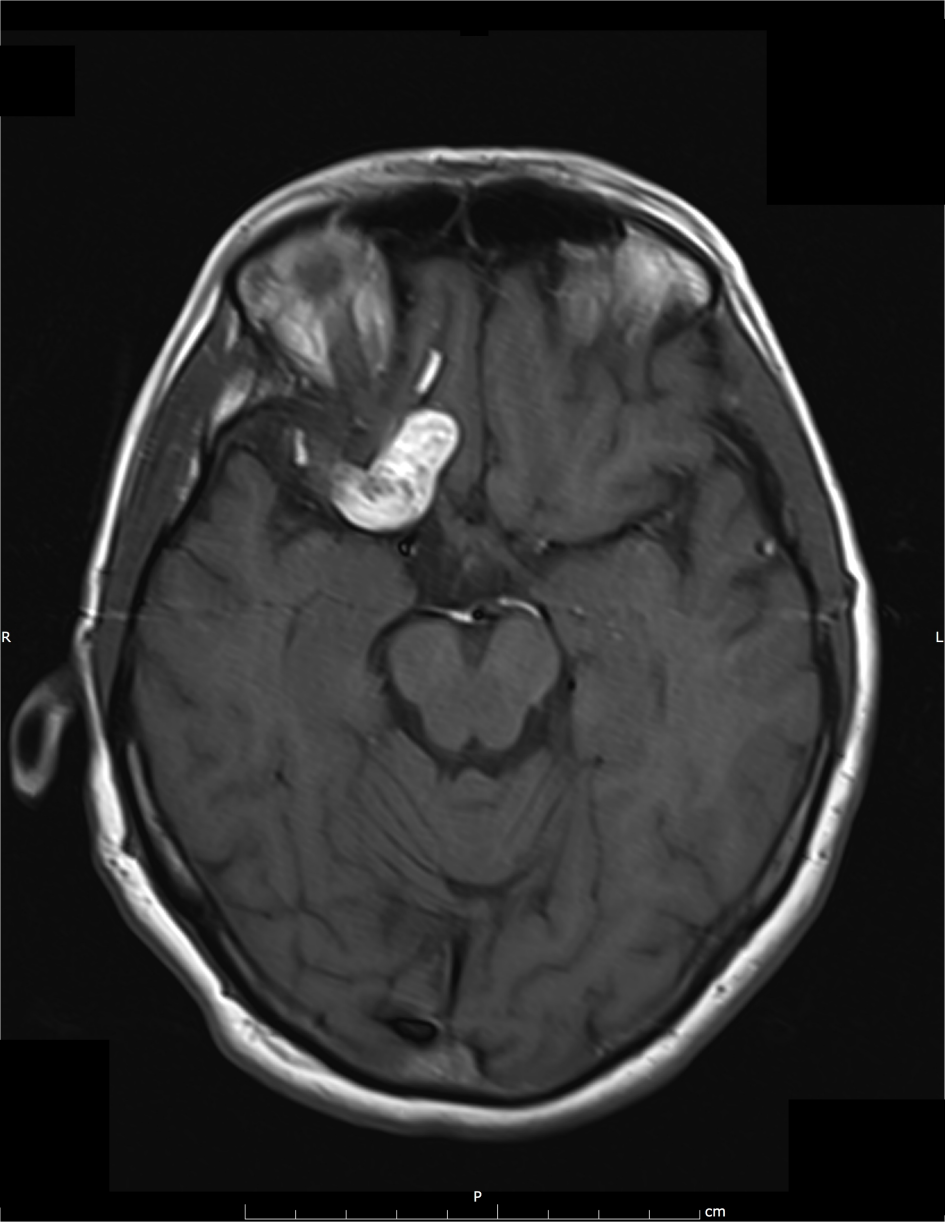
2008 Initial MRI This figure shows the MRI taken upon initial presentation in 2008. The image consists of a T1-weighted hyperintense mass in the right anterior cranial fossa. Also present are multiple small foci of T1-hypersensitivity within the right frontotemporal sulci suggesting prior rupture.
